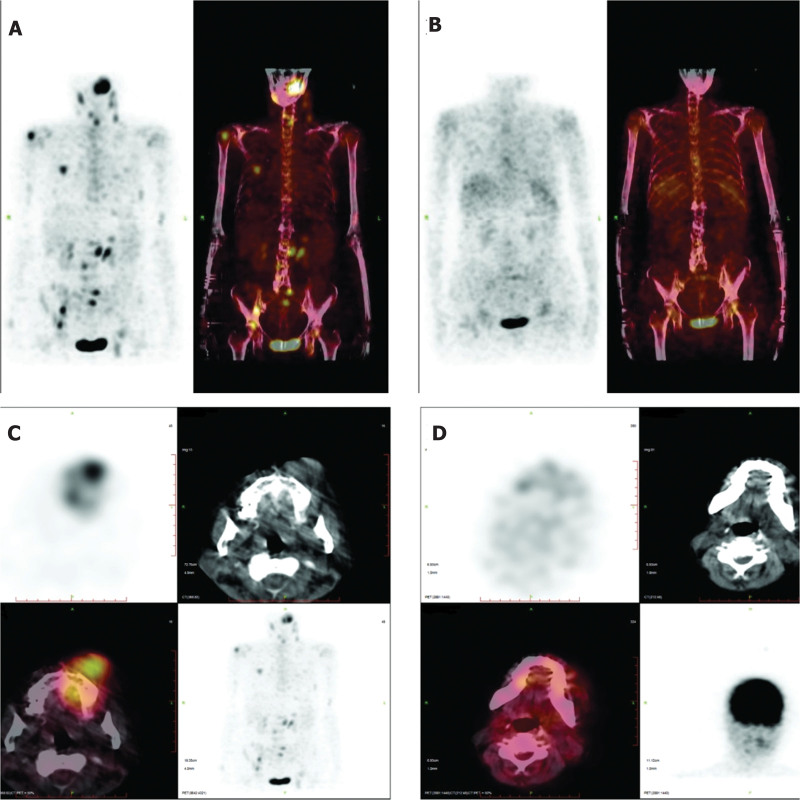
SPECT/CT report before and after treatment. (A) Pretreatment whole-body SPECT/CT report; (B) Post-treatment whole-body SPECT/CT report; (C) pretreatment localized SPECT/CT report; (D) Post-treatment localized SPECT/CT report.
